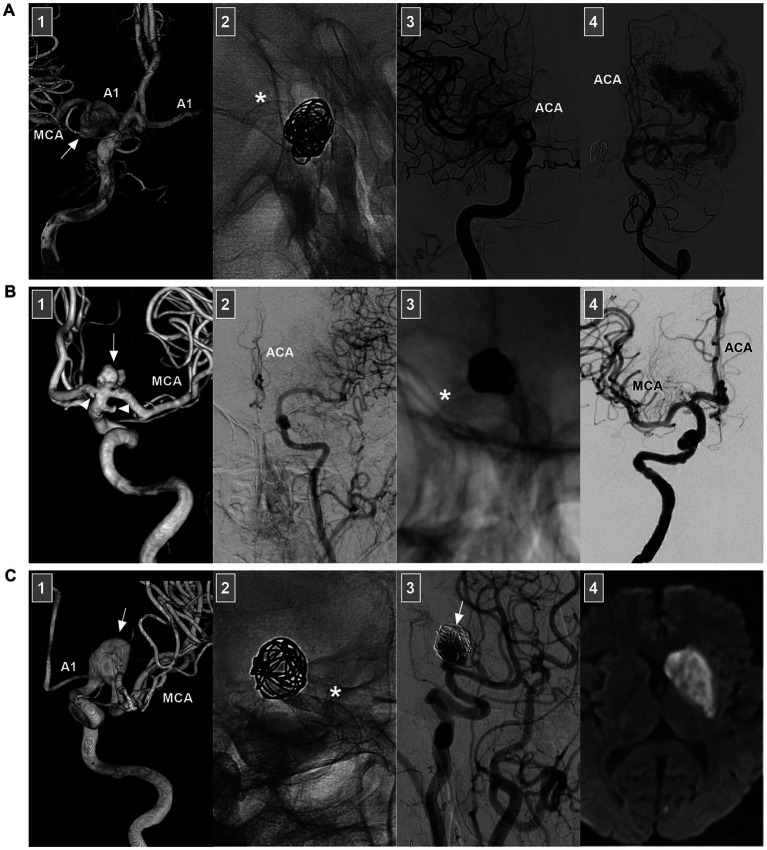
FD deployment of ICA bifurcation region aneurysms. (A) Number 1 panel: Three-dimensional DSA of the ICA showing an unruptured fusiform dissecting aneurysm (arrow) beyond the AchA involving the ICA terminus, the AcomA was patent, and the contralateral A1 can be seen. Number 2 panel: X-ray image showing the coils and FD deployment (asterisk). Number 3 panel: DSA of the ICA showing that the ipsilateral ACA was occluded. Number 4 panel: DSA of VA showing the arteriovenous malformation opposite the aneurysm; the ACA can be seen. Postoperatively, the patient had no symptom due to ACA occlusion. (B) Number 1 panel: Three-dimensional DSA showing an unruptured saccular aneurysm (arrow) at the ICA terminus; the associated A1 origin and AchA aneurysms (arrowheads) were found. Number 2 panel: DSA of the contralateral ICA showing the hypoplastic proximal ACA. Number 3 panel: X-ray image showing the coils and FD deployment (asterisk). Number 4 panel: Postoperative DSA showing that the ACA and MCA were patent. (C) Number 1 panel: Three-dimensional DSA showing an unruptured fusiform dissecting aneurysm (arrow) at M1 origin involving the ICA terminus. Number 2 panel: X-ray image showing the coils and FD deployment (asterisk). Number 3 panel: 6 months follow-up DSA of the ICA showing that the aneurysm (arrow) was occluded partially. Number 4 panel: After the transition to single antiplatelet agent, the patient suffered acute infarction of the caudate nucleus on magnetic resonance imaging. ACA, anterior cerebral artery; A1, first segment of the ACA; AchA, anterior choroidal artery; AcomA, anterior communicating artery; DSA, digital subtracted angiography; ICA, internal carotid artery; MCA, middle cerebral artery; VA, vertebral artery.
